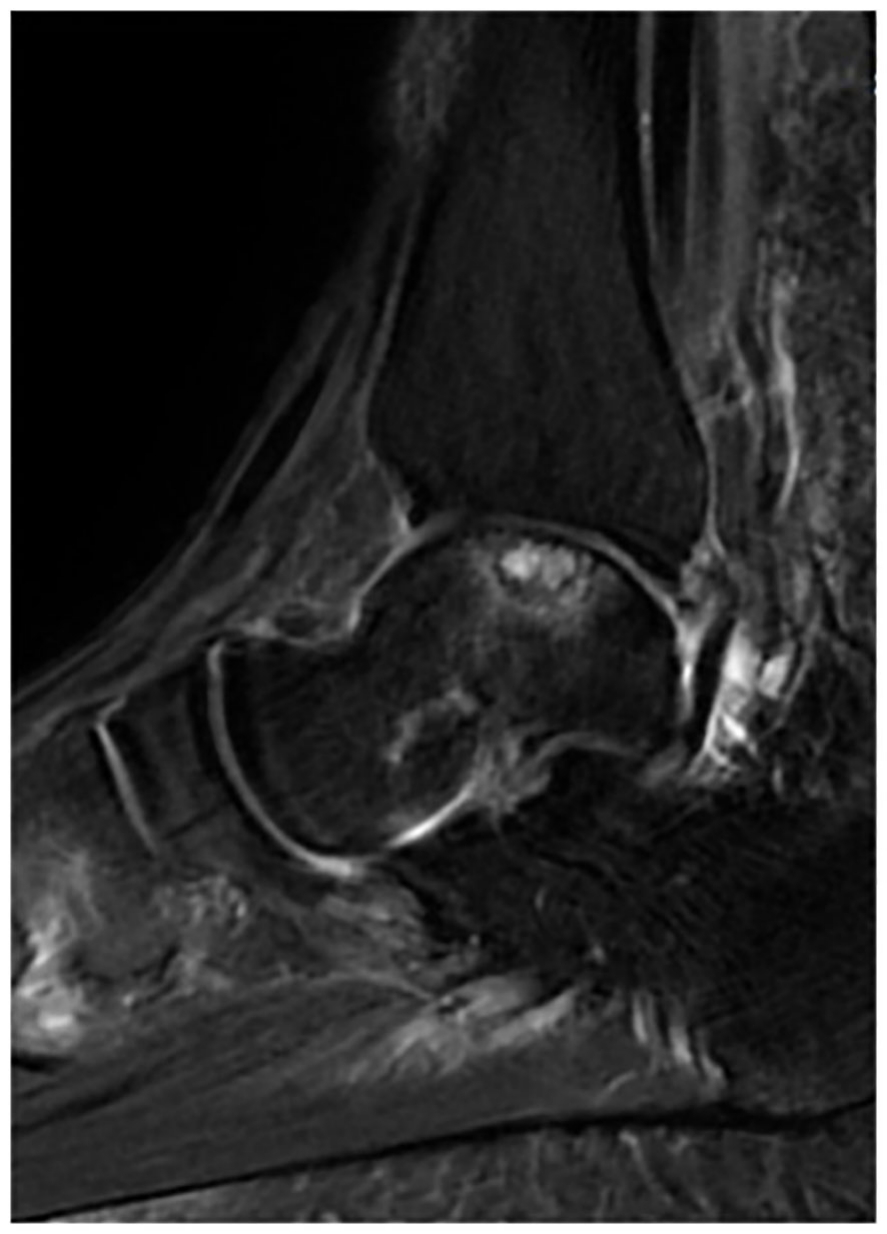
Patient’s preoperative ankle MRI. The patient’s preoperative MRI imaging data, which showed an area of high-density lesion under the talus cartilage, suggested the formation of a subchondral cyst, and the diagnosis of Hepple V OLT was made.
